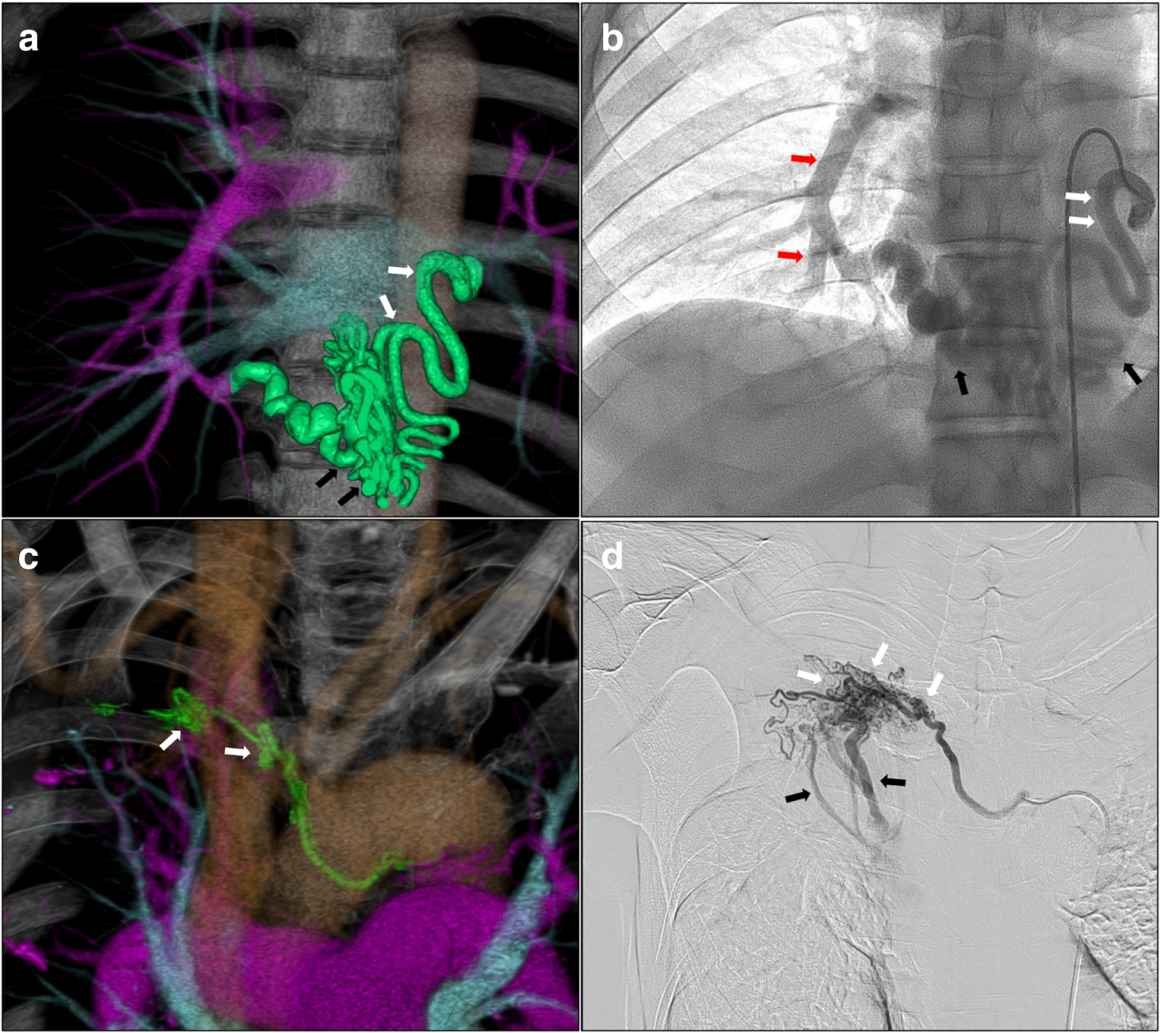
Three-dimensional CT (a, c) and angiography (b, d) findings before BAE. a Abnormal pulmonary ligament artery with vessel dilation (white arrows) and tortuosity (black arrows). b Vessel dilation (white arrows), tortuosity (black arrows), and systemic artery-pulmonary artery direct shunting (red arrows). c Abnormal bronchial artery with hypervascularity (white arrows). d Hypervascularity (white arrows) and systemic artery-pulmonary artery direct shunting (black arrows)
